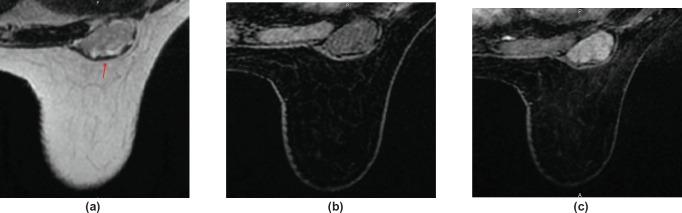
A 56-year-old woman with neurofibroma of the right sulcus: (a) T2W axial MR images demonstrate the mass is heterogeneously hyperintense compared to muscles and hypointense compared to fat tissues. The mass is within the breast but seated under the muscular aponeurotic fascia (arrow). (b) and (c) Axial T1W images with fat suppression; before gadolinium injection, the mass appears hypointense, with well-defined margins; (c) after gadolinium injection, the mass shows a heterogeneous enhancement.
